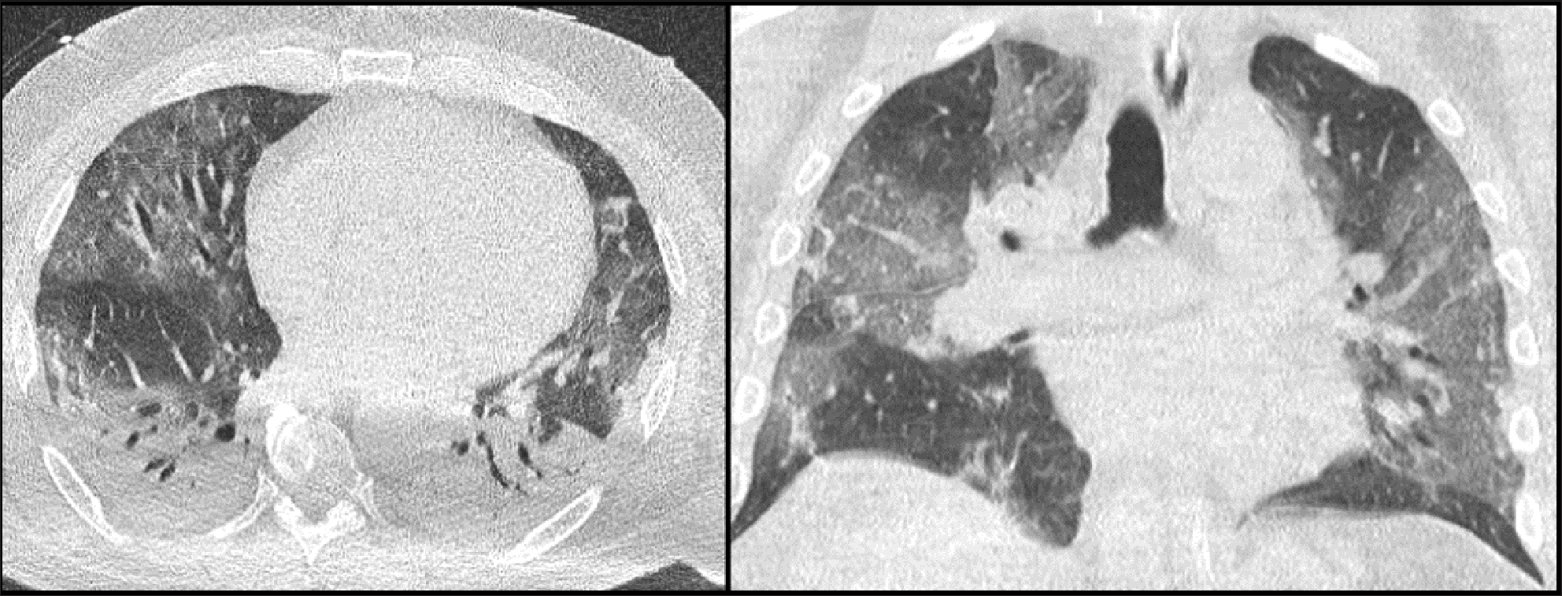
Low-dose chest CT with findings inconclusive for COVID-19 pneumonia, showing bilateral ground-glass opacities with peripheral-central distribution as a typical finding and bilateral pleural fluid as an atypical finding. RT-PCR and BAL turned out positive for COVID-19 in this patient.
